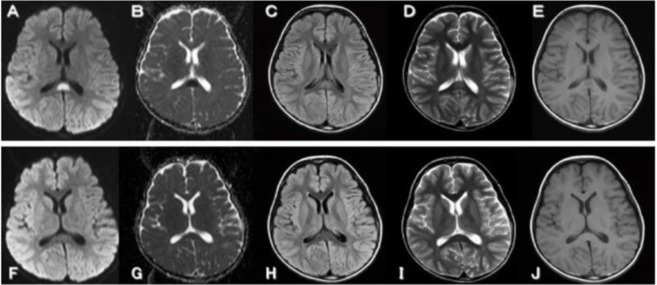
Brain magnetic resonance (MR) imaging on day 7 from the onset, showed abnormal signal intensities in the splenium of the corpus callosum: markedly high signal intensities on diffusion-weighted imaging (DWI) : (A), and markedly low signal intensities on an apparent diffusion coefficient (ADC) map : (B), slight hyperintensity developed in the same area on fluid-attenuated inversion recovery (FLAIR) : (C: TR=9000 / TE=105) and on fast spin-echo T2-weighted image : (D: TR=3800 / TE=99). Otherwise on spin-echo T1- weighted images showed mild decreased intensity signal in the splenium: (E: TR=593 / TE=15). MR imaging findings on day 13, demonstrating completely resolved of these abnormal intensities on DWI: (F), ADC map: (G), FLAIR : (H), T2-weighted image : (I) and T1-weighted image : (J)
